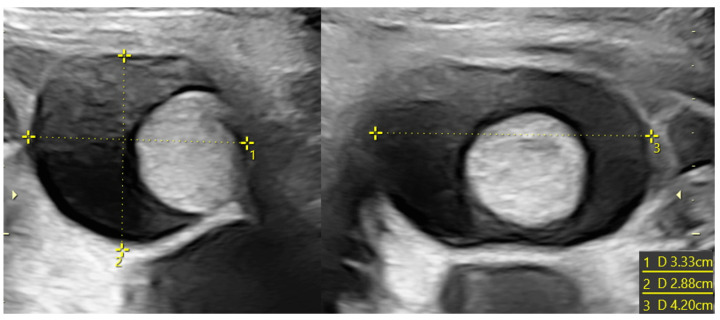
Fetal ovarian cyst at 35 weeks of gestation described as mixed in its content (42 × 33 × 29 mm). The central rounded formation, presumably a blood clot, was not vascularized at Power Doppler, and at postnatal follow-up evaluations, the cyst progressively decreased until disappearing within a month.
